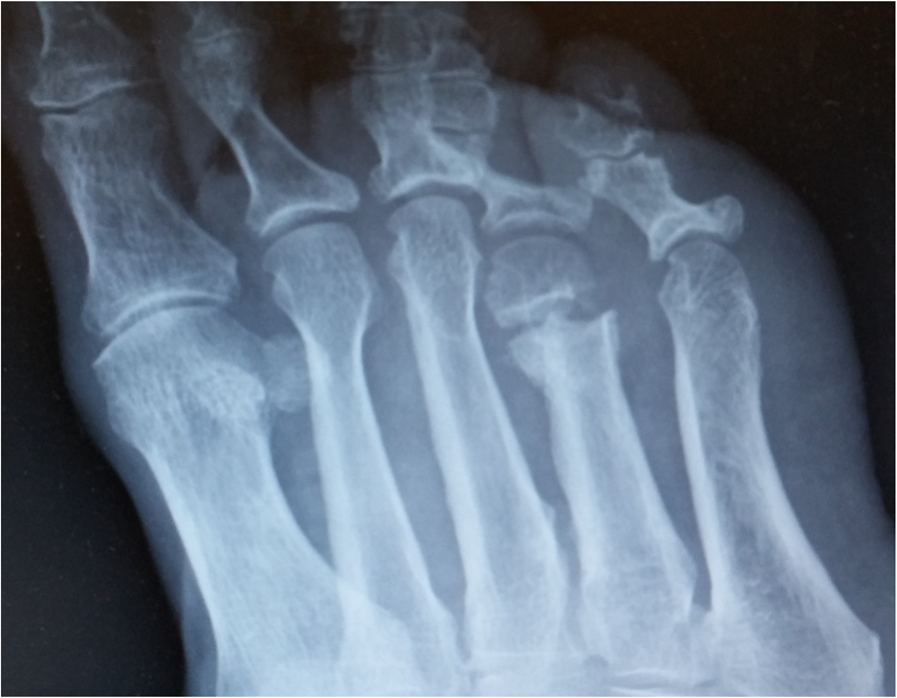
Minimally invasive floating metatarsal osteotomy. Post-operative x-ray demonstrating an osteotomy of the neck of the 4th metatarsal
